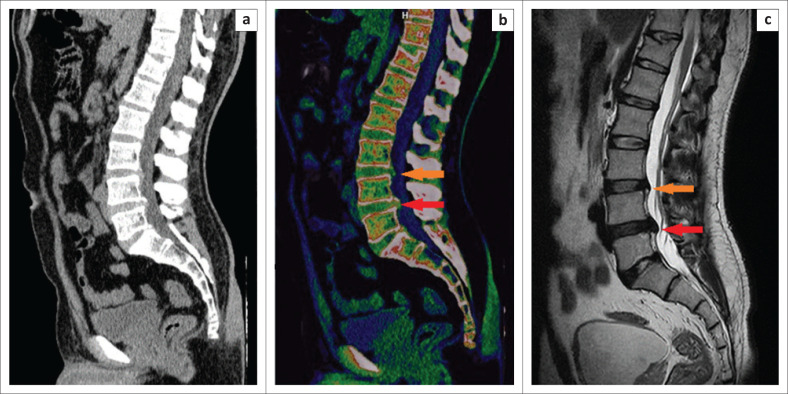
A 40-year-old man who presented with chronic lower back pain with recent aggravation: (a) The lumbar disc protrusions at L3/L4 and L4/L5 levels were initially not clearly depicted on the sagittal grayscale standard CT reconstructions. (b) The lumbar disc protrusions (orange and red arrows) depicted on the sagittal colour-coded electron-density reconstruction images optimised for analysis of intervertebral discs. (c) Non-contrast sagittal T2-weighted MRI confirmed the diagnosis.
